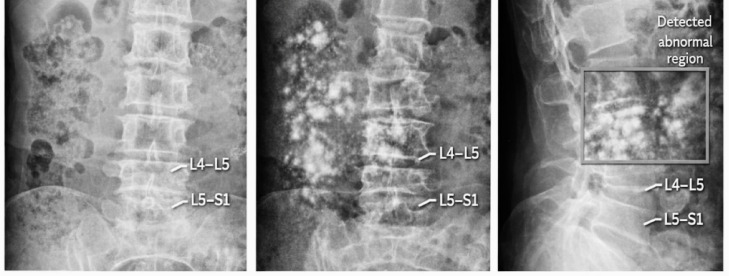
Visualization of structural variations and abnormal region detection in lumbar spine radiographs.
